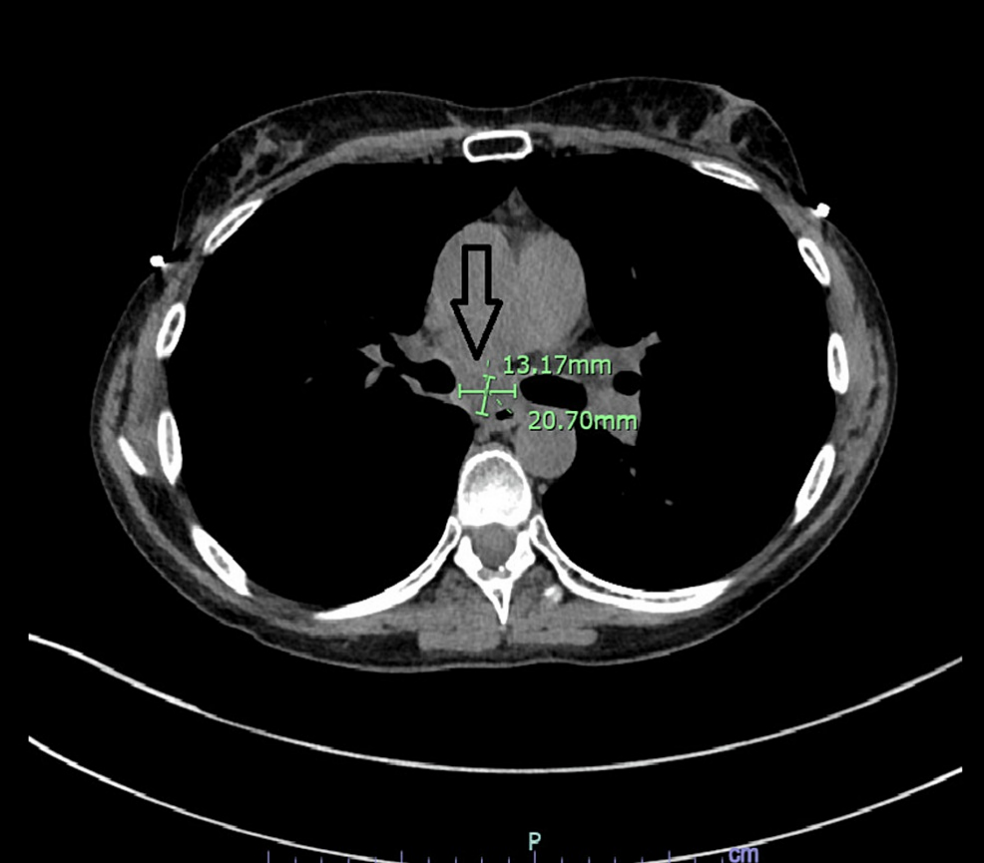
Followup CT chest A follow-up CT scan of the chest showed a slightly reduced size of the subcarinal mass (arrow).
